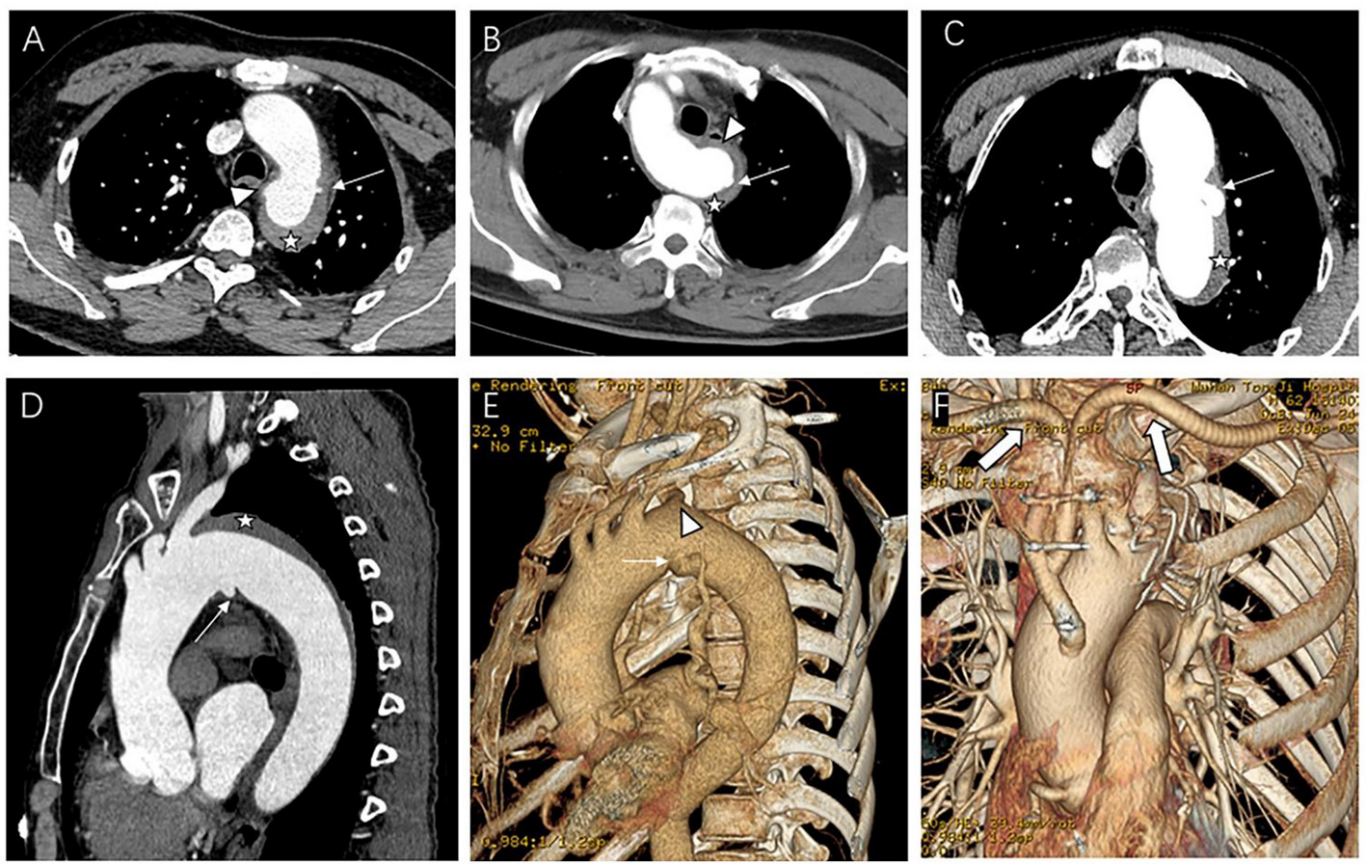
Contrast-enhanced CT angiography (CTA) image of the aberrant subclavian artery patients with aortic intramural hematoma (IMH) and penetrating atherosclerotic ulcer (PAU) or ulcer-like projection (ULP). (A) Patient with PAU (arrow) located near the aberrant right subclavian artery (ARSA) ostium (arrowhead) and IMH (star); (B) Patient with aberrant light subclavian artery and IMH (star), the PAU (arrow) located on the Kommerell's diverticulum (KD) (arrowhead). (C–F) Patient with ULP (thin arrow) located near the ARSA ostium (arrowhead) and IMH (star) underwent hybrid repair, thick arrow showing the artificial blood vessel from ascending aorta to subclavian arteries.
